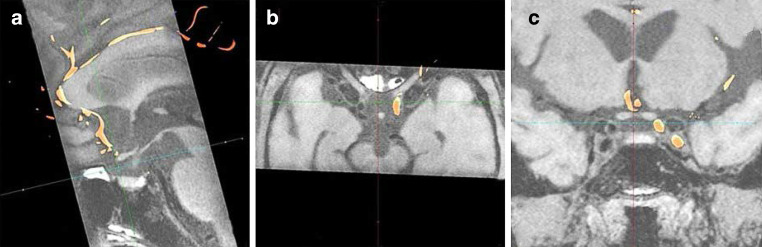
Fusion results of three-dimensional digital subtraction angiography (3D DSA) and magnetic resonance imaging (MRI). The 3D DSA and MRI are fused using a machine-based technique on the three images automatically displaying in real-time. The 3D DSA (*orange*) and MRI (*background*) fusion results of the major arteries are seen in the same window in a sagittal, b coronal and c axial orientations
